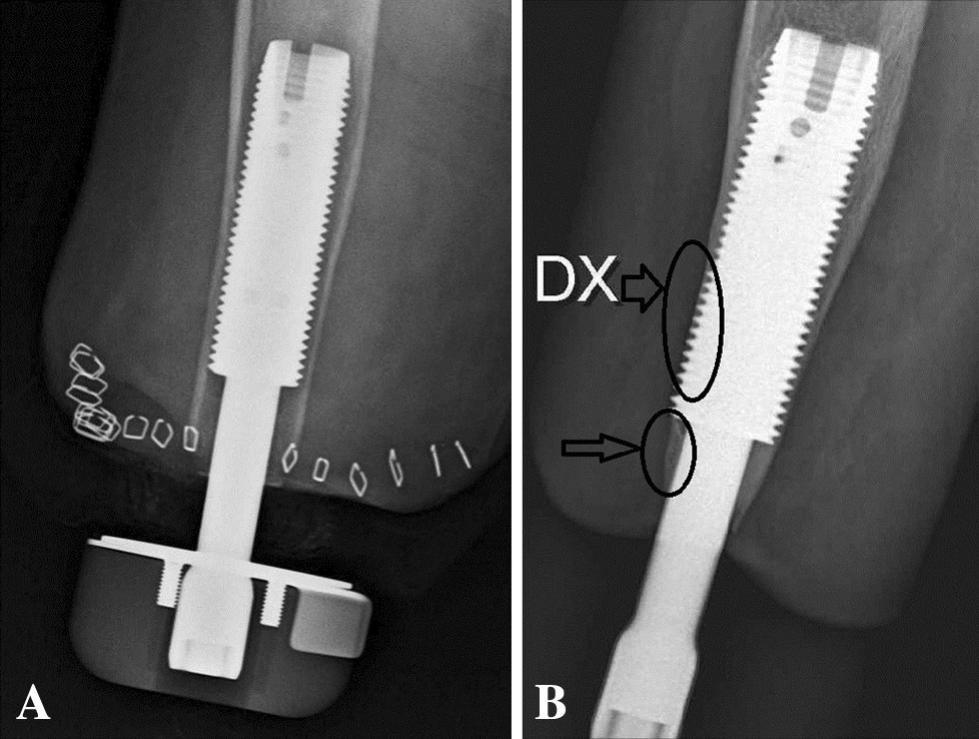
This radiograph at 3-year followup (B) shows signs of cortical thinning in the distal third of the fixture (upper arrow) and distal bone resorption at the bone canal distally to the fixture (lower arrow). Some proximal buttressing is also evident at the proximal third of the fixture. Comparison is made to the S2 postoperative radiograph (A).
